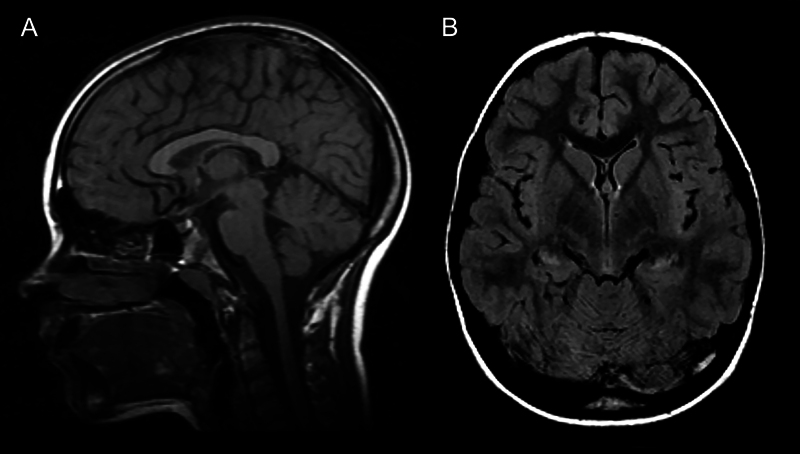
Wouter was born after a planned home delivery at 40 weeks gestational age with a birth weight of 4,900 gram, and a poor start (Apgar 0/1/2 at 1, 5, and 10 minutes) due to a shoulder dystocia. He was admitted to the NICU and developed seizures, which were treated with phenobarbital, phenytoin, and midazolam. Besides a small subdural hemorrhage, no other abnormalities were noted on an MRI performed on day 10. At 24 months, the Griffiths Mental Developmental Scales (GMDS) score demonstrated normal neurodevelopment (locomotor 26 months, personal–social 24 months, hearing–speech 24 months, eye–hand 24.5 months, and performance scale 23.5 months). Brain MRI performed at 10 years of age demonstrated mammillary body atrophy on sagittal T1-weighted imaging (
